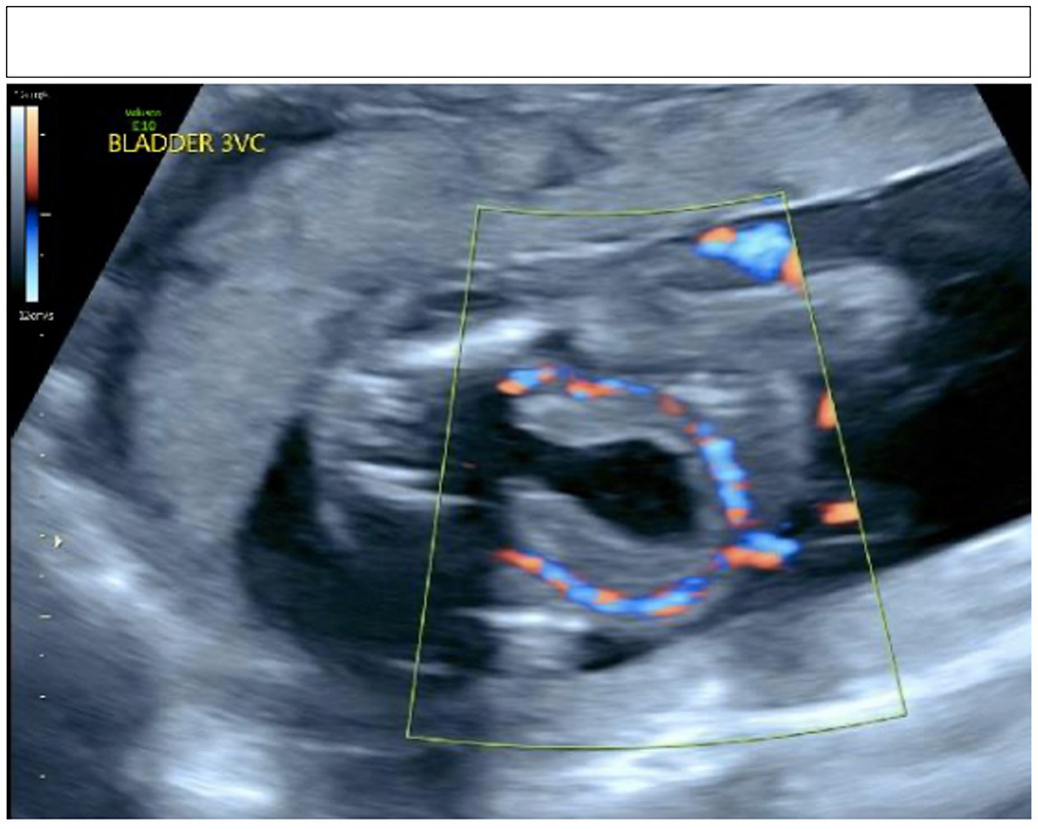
Keyhole sign on prenatal ultrasonography.
